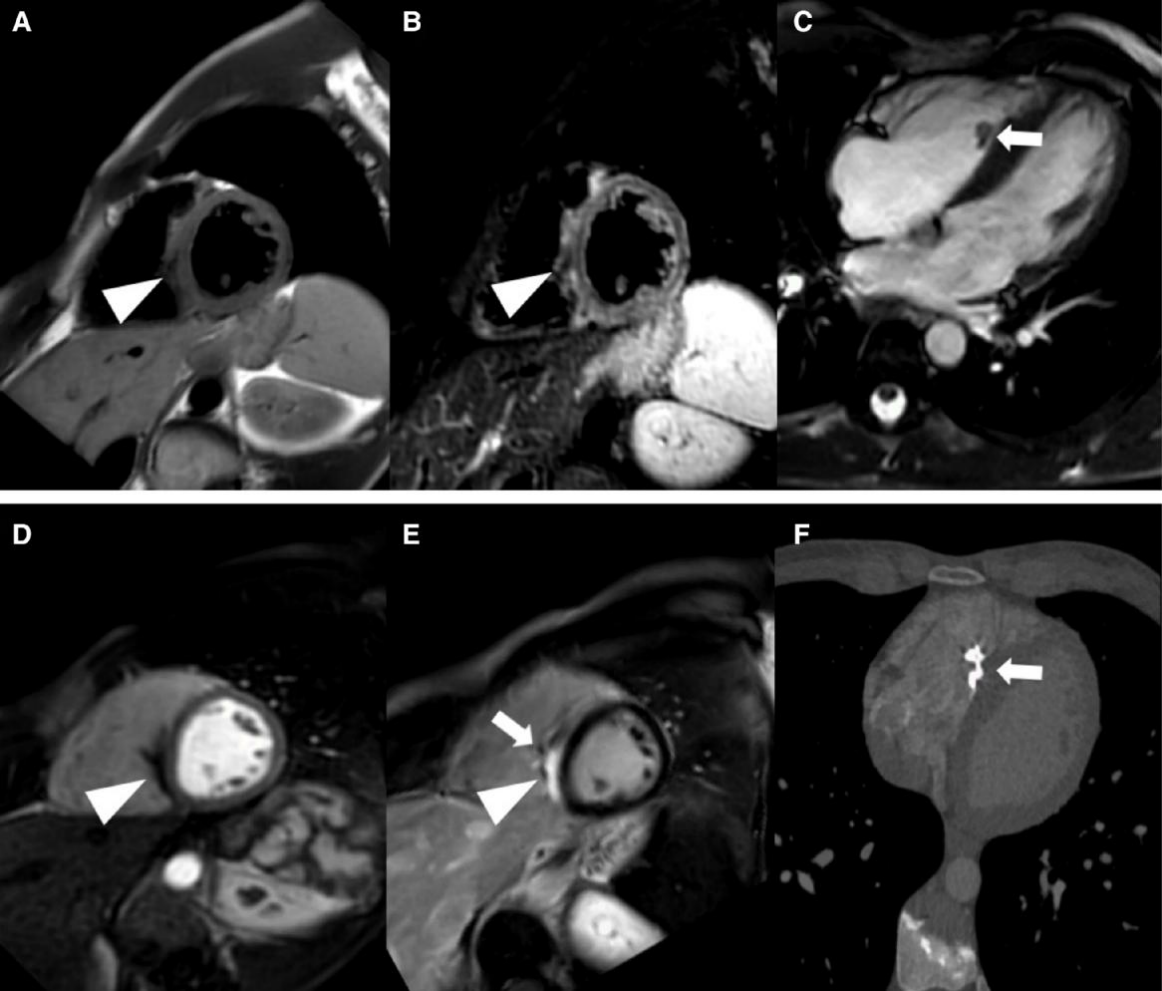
Cardiovascular magnetic resonance showing a broad-based cardiac mass of the right ventricular septal wall with an iso- to hypointense signal in T1- (*A*, arrowhead) and hypointense signal in T2-weighted (*B*, arrowhead) sequences in short axis. Cine sequence in four-chamber view revealed an iso- to hypointense signal (*C*, arrow). There was no hypervascularization of the mass first-pass-perfusion study in short axis (*D*, arrowhead). Late gadolinium enhancement revealed a homogeneous enhancement in short axis (*E*, arrowhead) with adjacent hypointense structures (*E*, arrow). Subsequent CT revealed corresponding dystrophic calcifications in this position (*F*, arrows). Overall, these features are consistent with a cardiac fibroma.
